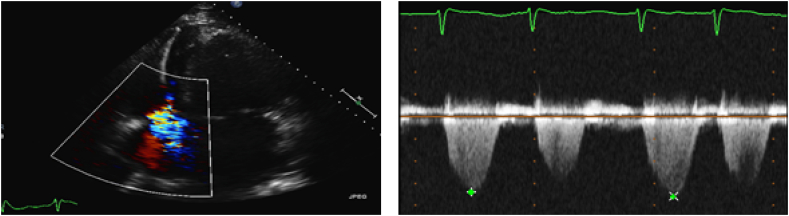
Color & spectral Doppler showing moderate to severe TR. (For interpretation of the references to colour in this figure legend, the reader is referred to the Web version of this article.)
